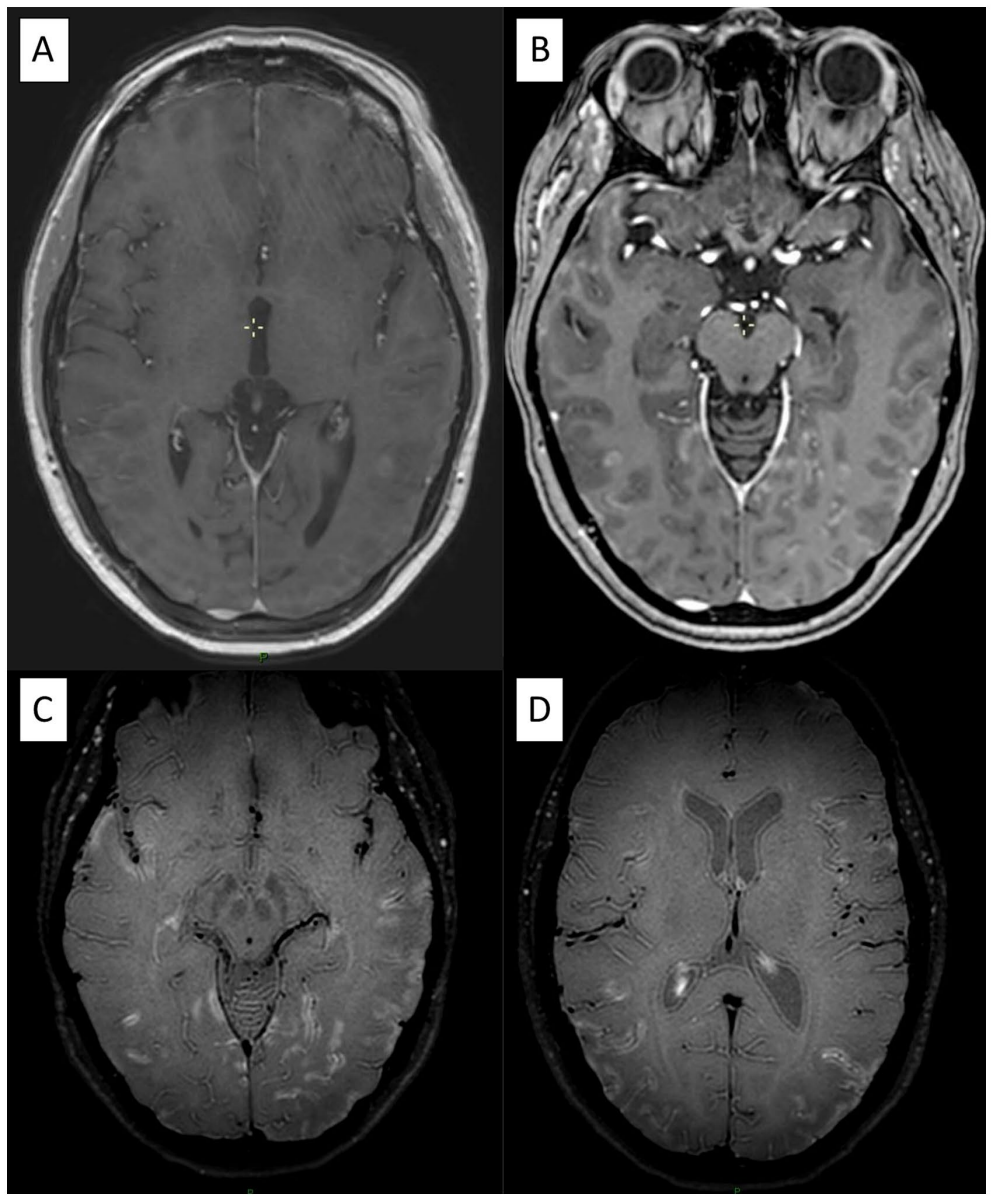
A 40-year-old patient with biopsy-proven small vessel vasculitis. (A) Post-gadolinium 3D T1-weighted MRI shows parenchymal enhancement in the parietal lobe and associated leptomeningeal enhancement (B). (C and D) VWI demonstrates diffuse and extensive leptomeningeal contrast enhancement.
